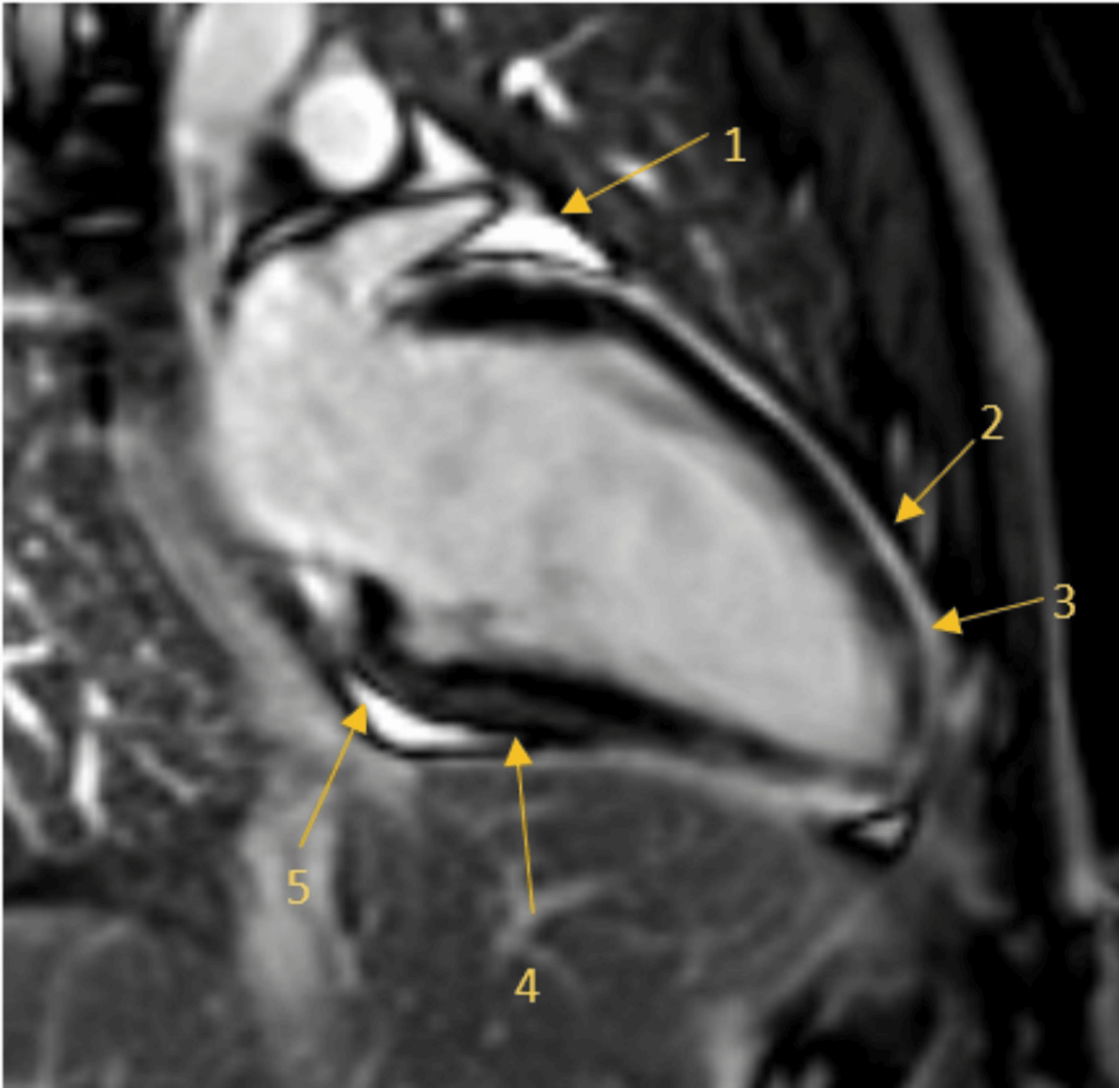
CMRI showing two-chamber view demonstrating pericardial fluid and LGE Arrow 1: localised pericardial fluid in the anterior segment; Arrow 2 + 3: patchy LGE in the apical anterior segment; Arrow 4: basal and mid inferior segment LGE; Arrow 5: localised pericardial fluid in basal segment LGE: late gadolinium enhancement; CMRI: cardiac magnetic resonance imaging
